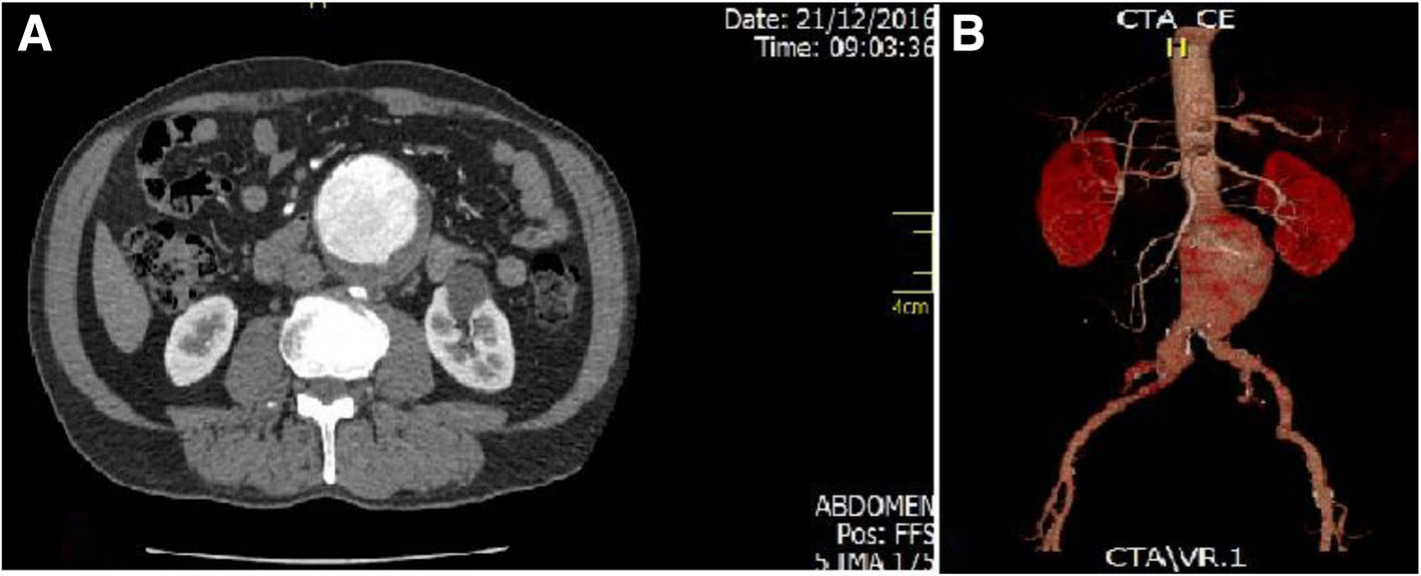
a Abdominal computed tomographic (CT) scan in transverse section prior to endovascular surgery. An infrarenal aortic aneurysm is observed. b CT angiography showing infrarenal aortic aneurysm
